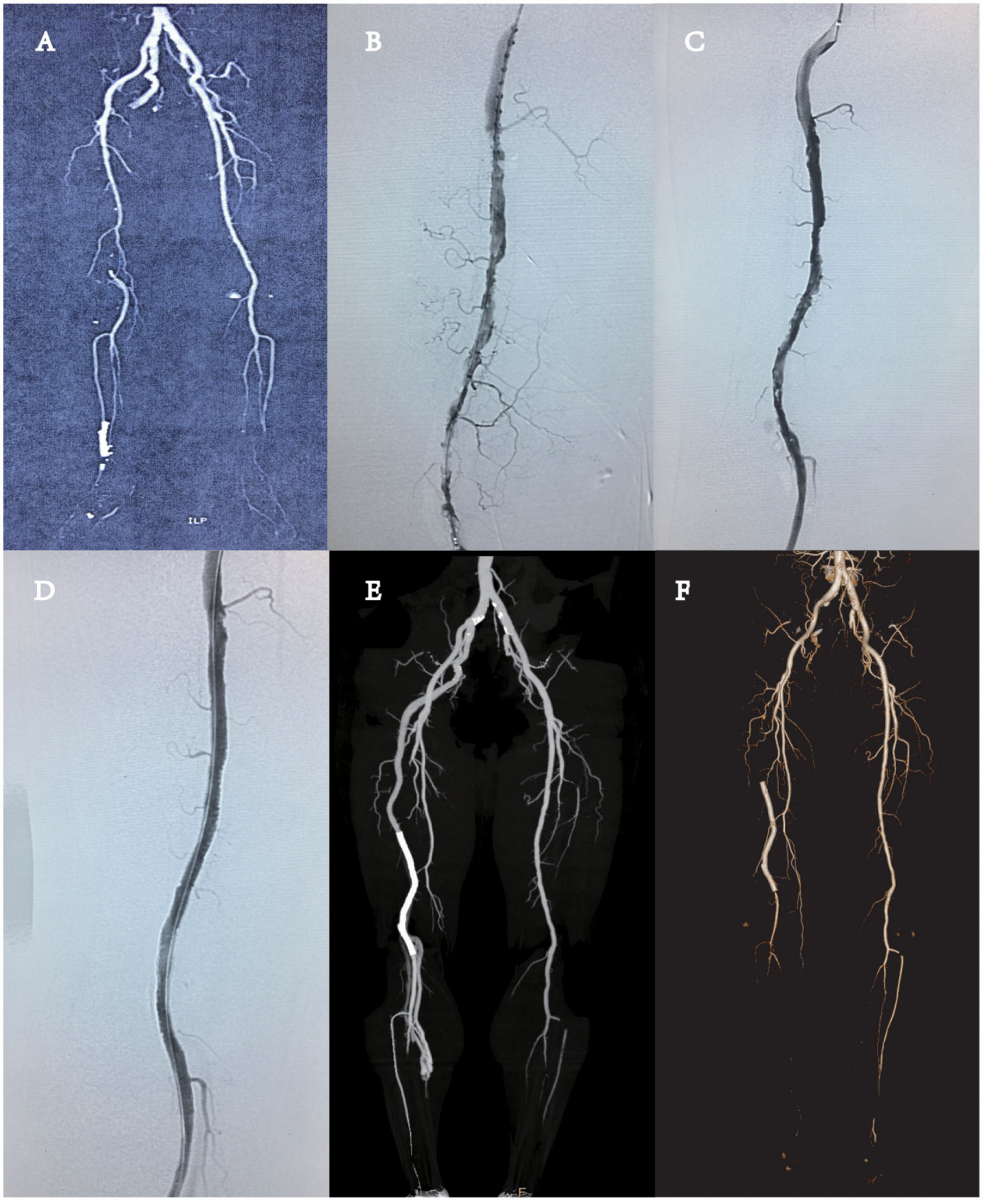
(A) Computed tomography angiography (CTA) showed the presence of complete persistent sciatic artery (PSA) with an incompletely developed superficial femoral artery (SFA) (type 2A PSA), without any aneurysmal change, and the complete obstruction of near-end popliteal artery by thrombus. (B) DSA revealed the obstruction of the remote of PSA and popliteal artery by thrombus. (C) One day of thrombolysis digital subtraction angiography (DSA) examination revealed partial dissolution of the thrombus in the blood vessels and multiple stenoses in the lumen, and advanced thrombosis was considered. (D) DSA revealed the vascular stent patency and no stenosis and thrombosis. (E) CTA revealed the patency of the whole PSA. There was no thrombosis or stenosis in the vascular stent. (F) CTA revealed that thrombosis in the vascular stent and the vessel was occluded.
